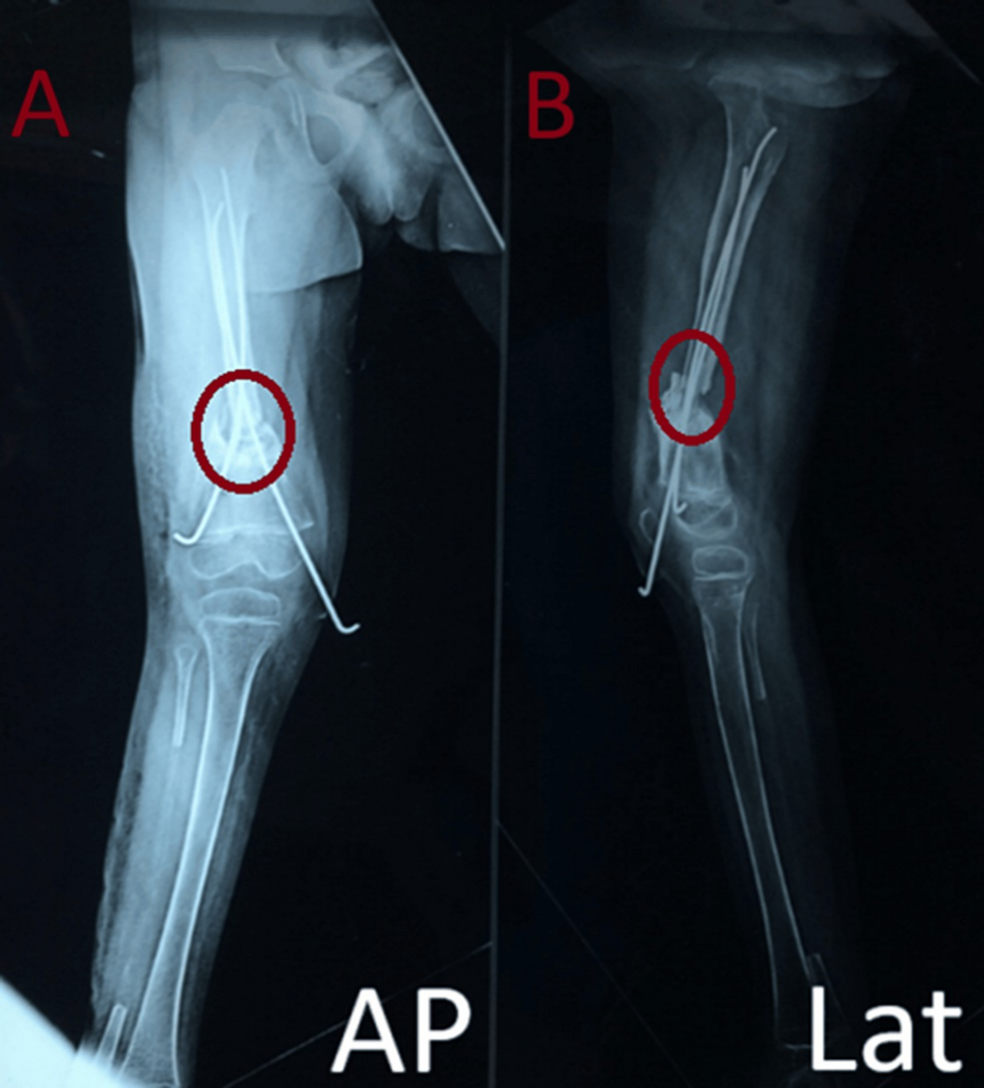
Second-month follow-up radiographs showing progressive signs of fracture union A: AP view, B: Lateral view
